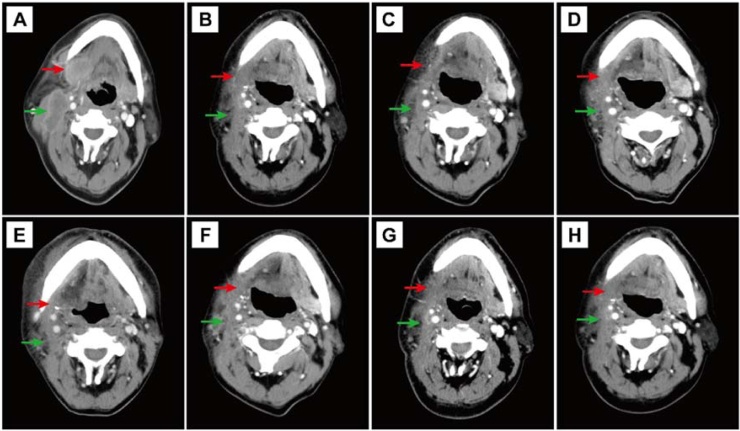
Contrast-enhanced CT scans demonstrating the tumour (20 × 25 × 32 mm) located in the right submandibular gland area (red arrow) and multiple enlarged lymph nodes (green arrow) in the right neck (A). Follow-up contrast-enhanced CT scans at 1-month (B), 6-months (C), 12-months (D), 15-months (E), 21-months (F), 27-months (G), and 36-months (H).
